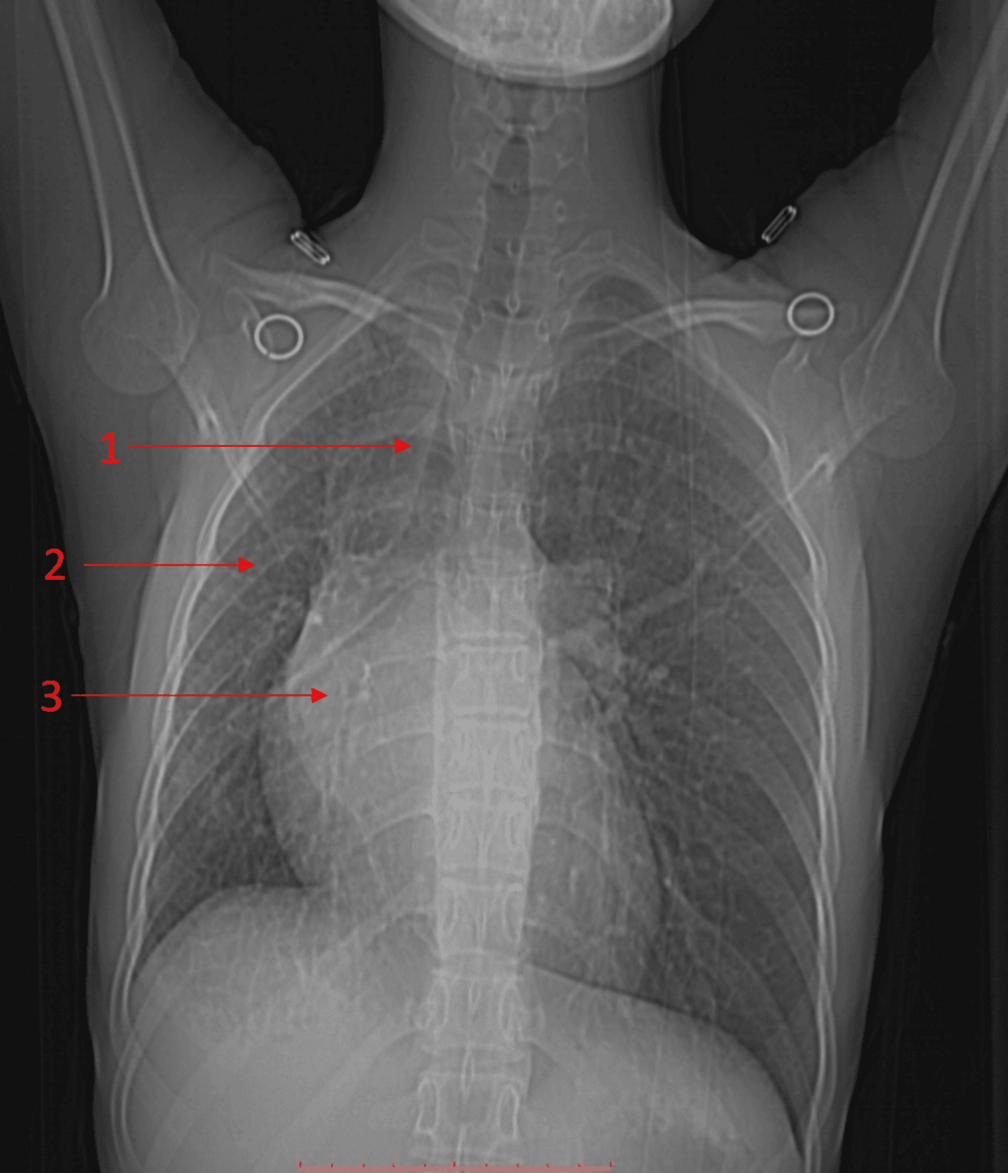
Case 1: X-ray and scout film 1 indicates the tracheal and mediastinal deviation to the right side. 2 indicates the volume loss of the right lung. 3 indicates the obscured right hilar shadow.
